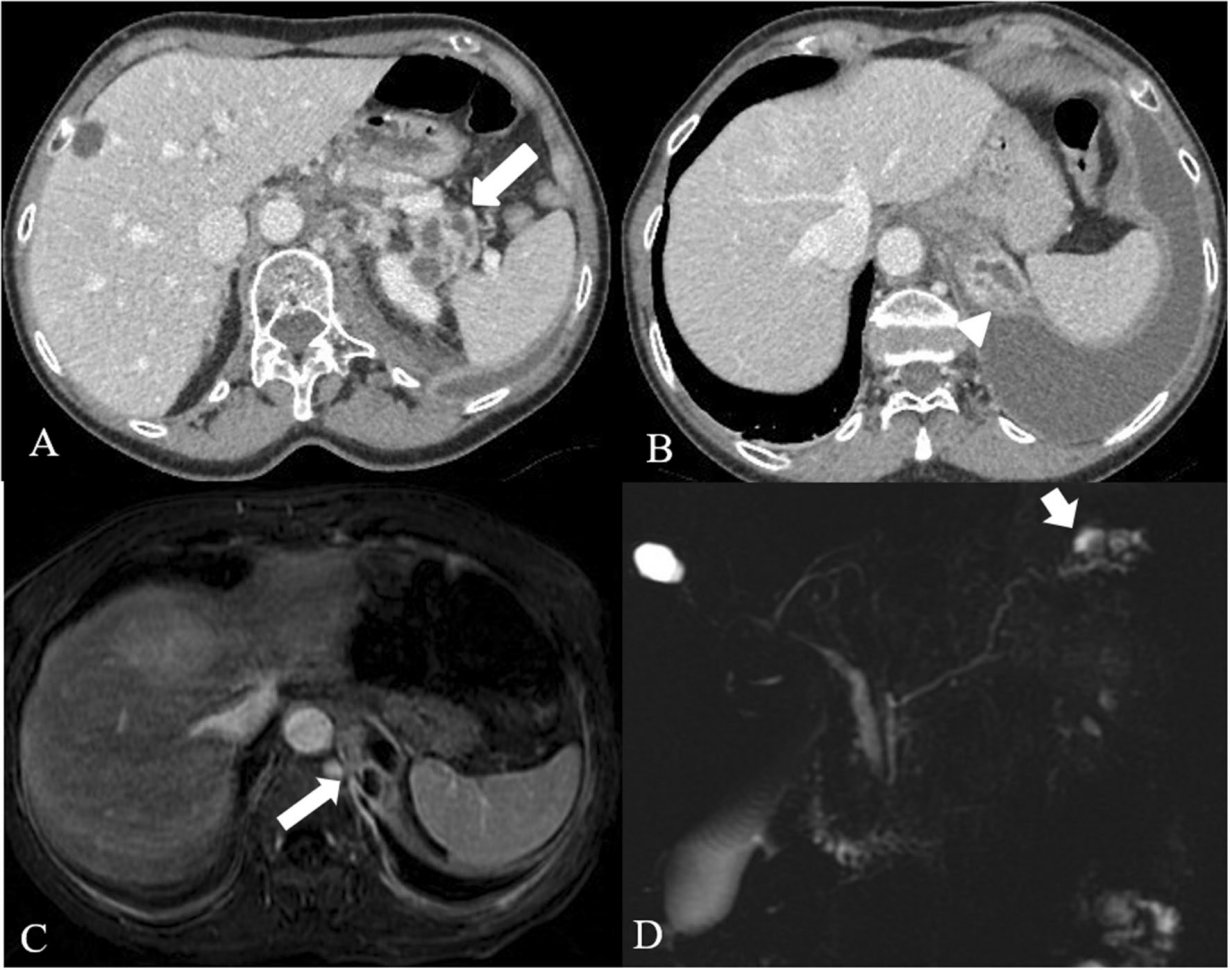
Fistula with pleural cavity in a 66 years-old woman with acute necrotizing pancreatitis. Multiple encapsulated fluid collections in the tail of the pancreatic parenchyma (arrow in a), as for necrotic spots. These collections extend upt to the left hemidiaphragm with pleural fistula and pleural effusion (arrowhead in b). Axial (c) post contrast T1w MR images and MRCP (d) confirm the pleural fistula (arrows)
